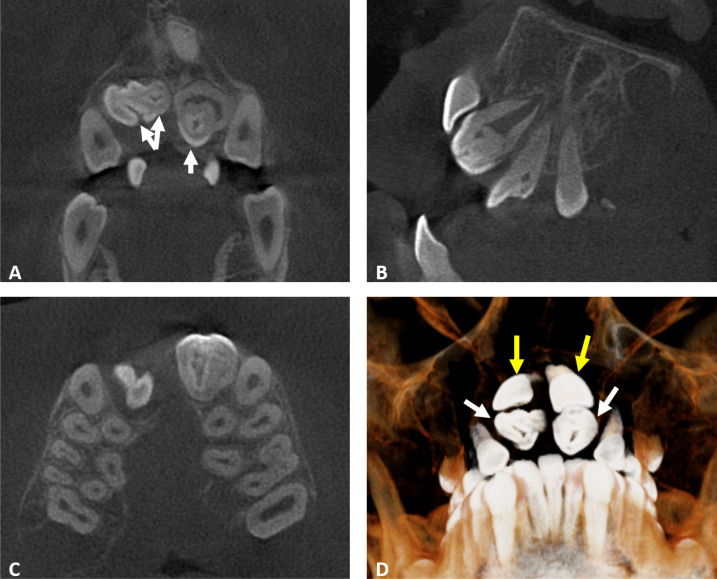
Coronal, sagittal, axial, and cross-sectional CBCT images that are examined. (A) Coronal CBCT section showing the presence of double invaginatus in 11 and enamel lined invaginatus of the maxillary lateral incisors (white arrows). (B) Sagittal section revealing the presence of dens invaginatus Oehlers Type 1 in the maxillary lateral incisors. (C) Axial section showing the unilateral cleft palate on the right side. (D) Three-dimensional reconstruction showing the altered morphology of lateral incisors (white arrows) and the impacted central incisors (yellow arrows).
